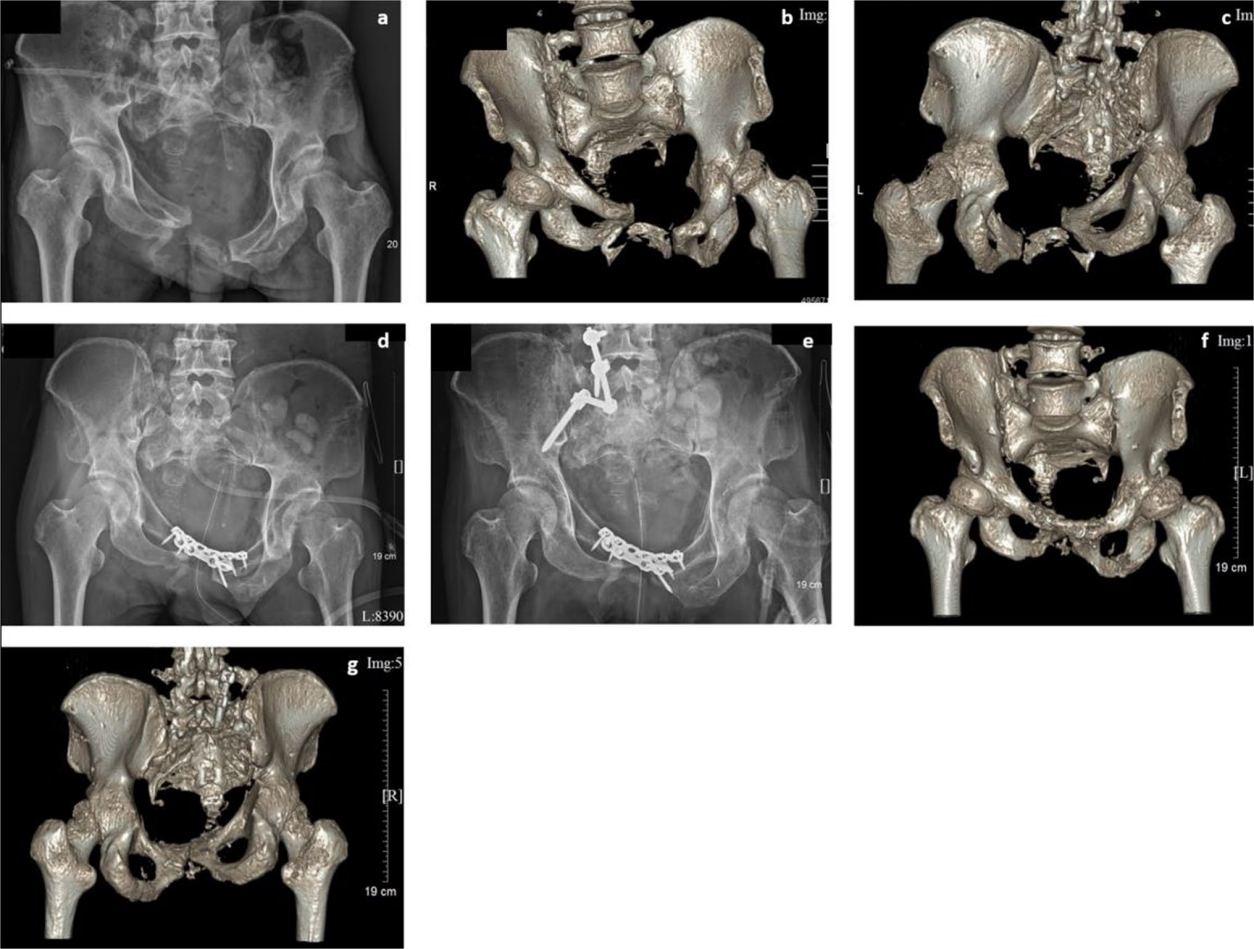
Male, 39 years old, 3 months after injury caused by a traffic accident, suffering from pain of the sacroiliac joint and pubic symphysis, with a bedsore on the sacrococcygeal region. a, b, c Post-operative X-ray and 3D reconstruction of CT scan showing posterosuperior sacroiliac joint dislocation and diastasis of the pubic symphysis. To avoid iatrogenic nerve injury and infection, we decided to perform a two-stage operation. d X-ray examination after the first stage, osteotomy of the posterior pelvic ring anteriorly through LRA without fixation; the anterior ring was fixed with reconstruction plates. e, f, g After 1 week of lower limb traction, the second stage was performed in the prone position; lumbopelvic distraction osteosynthesis was used in fixation
